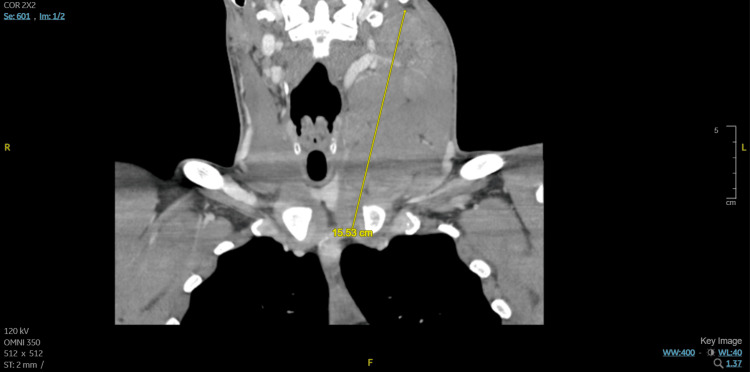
Computed tomography soft tissue neck with contrast with yellow line demonstrating enlarged left cervical lymph node conglomerate measuring 15.5 cm with significant mass effect
